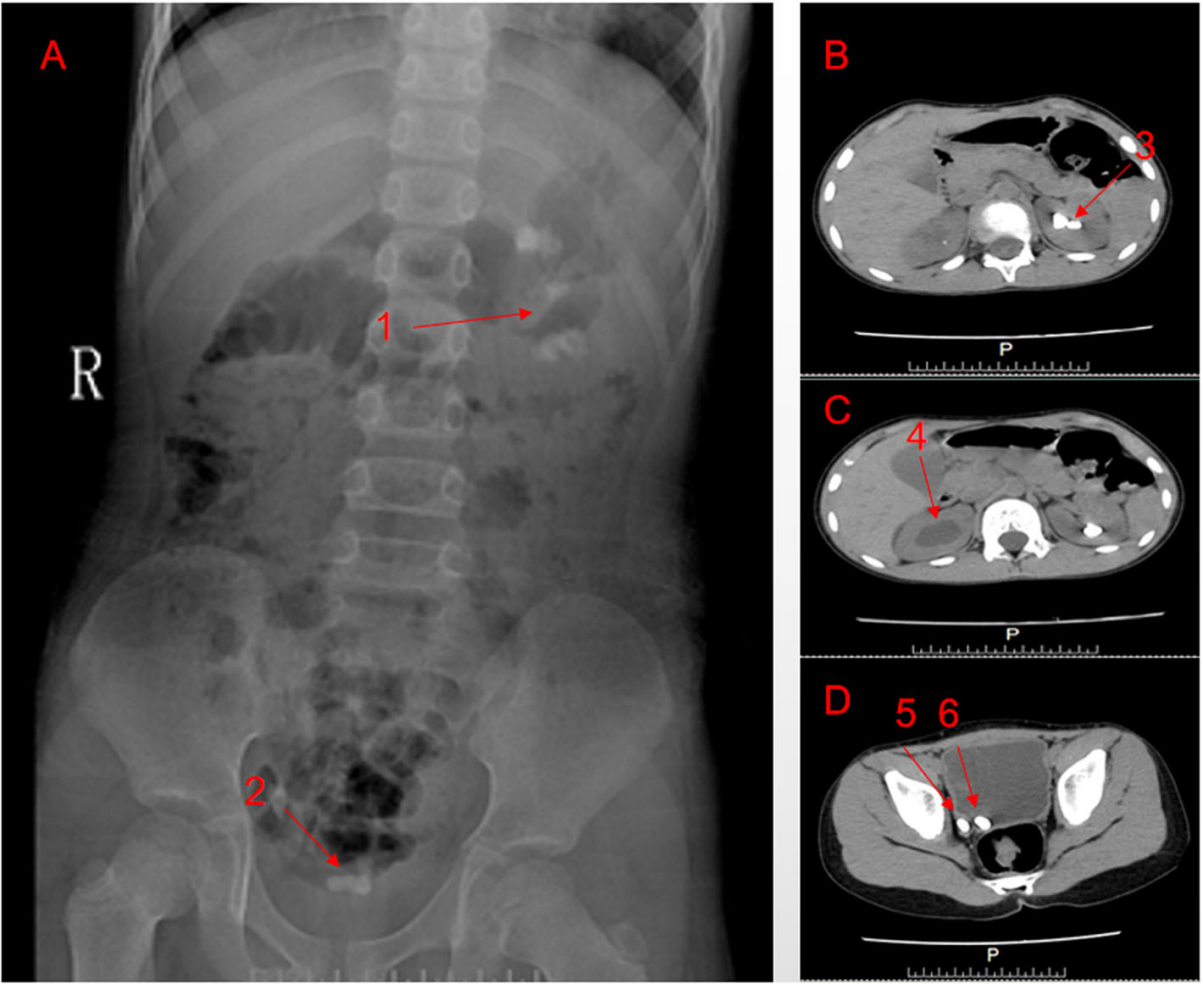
Radiographic findings in patient, the arrows indicate stones. a: KUB film, R indicates the right side of the body and the two red arrows indicate the stone detected in the kidney (arrow 1) and the bladder (arrow 2); b, c, d (CT axial image): b: the average CT value of arrow 3 is 1395.28HU; c: arrow 4 indicates hydronephrosis in the right kidney; d: the average CT values of arrows 5 and 6 are 1501.58HU and 1283.38HU respectively
